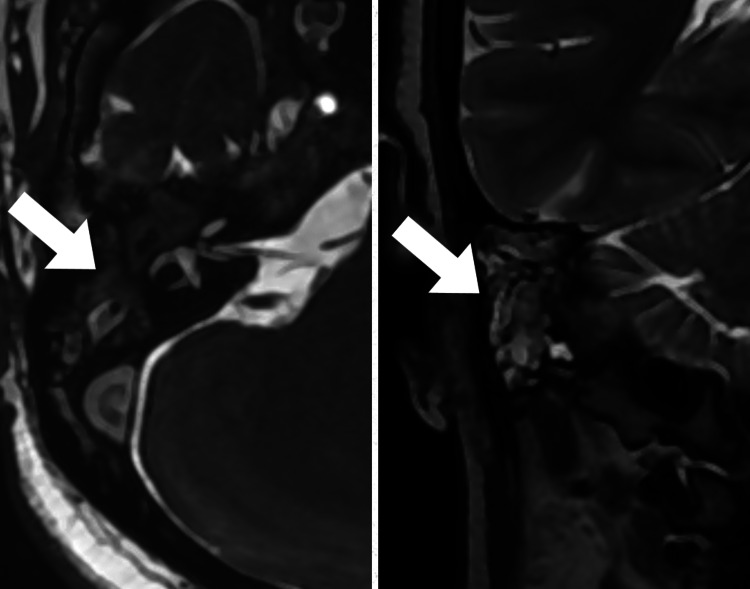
Axial and Coronal T2-weighted MR images show heterogeneously hyperintense foci within the right middle ear cavity and mastoid air cells. MR: magnetic resonance.
